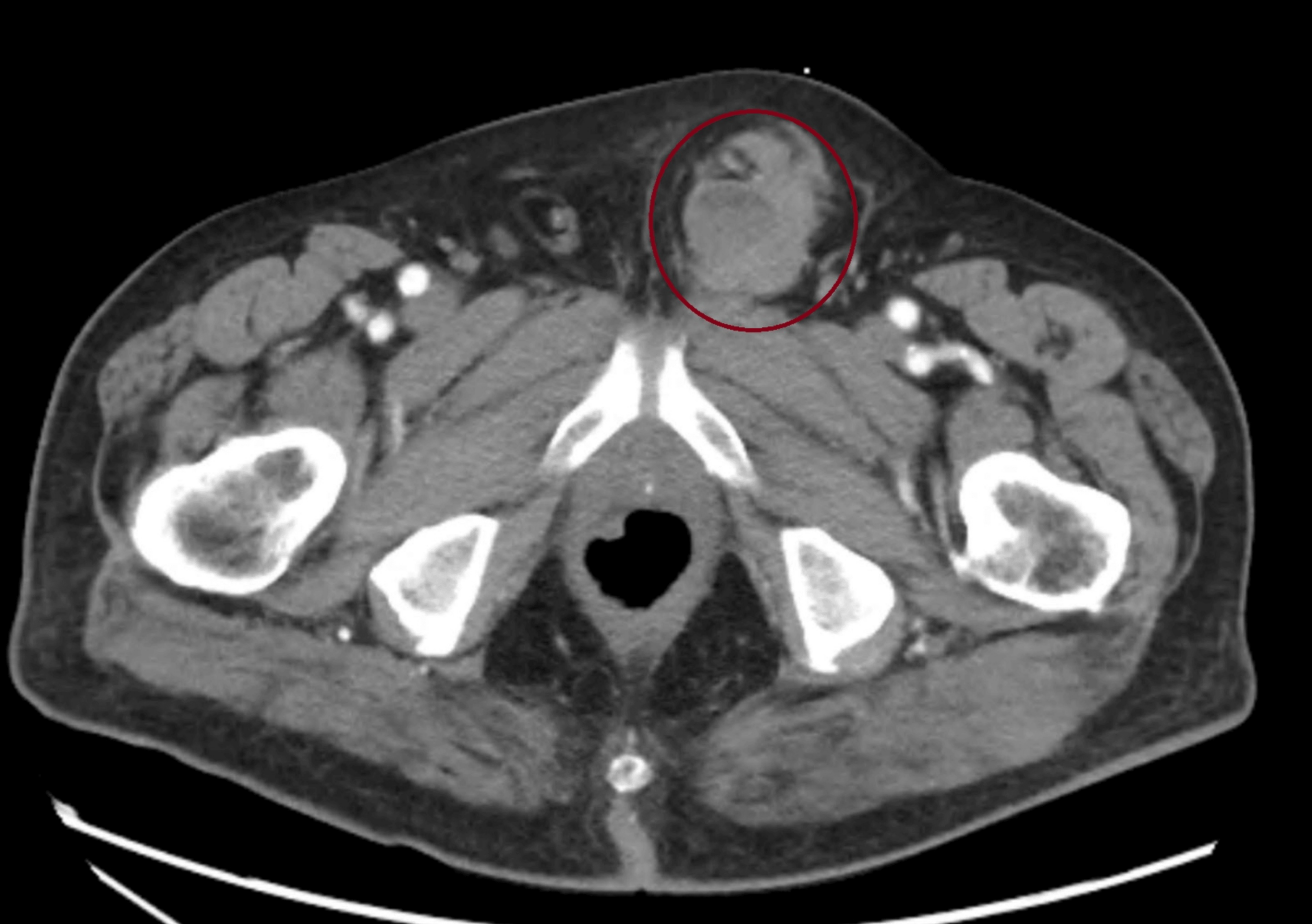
CT scan of the pelvis revealed a soft tissue mass over the left pubic tubercle, suggestive of a left inguinal hernia.
